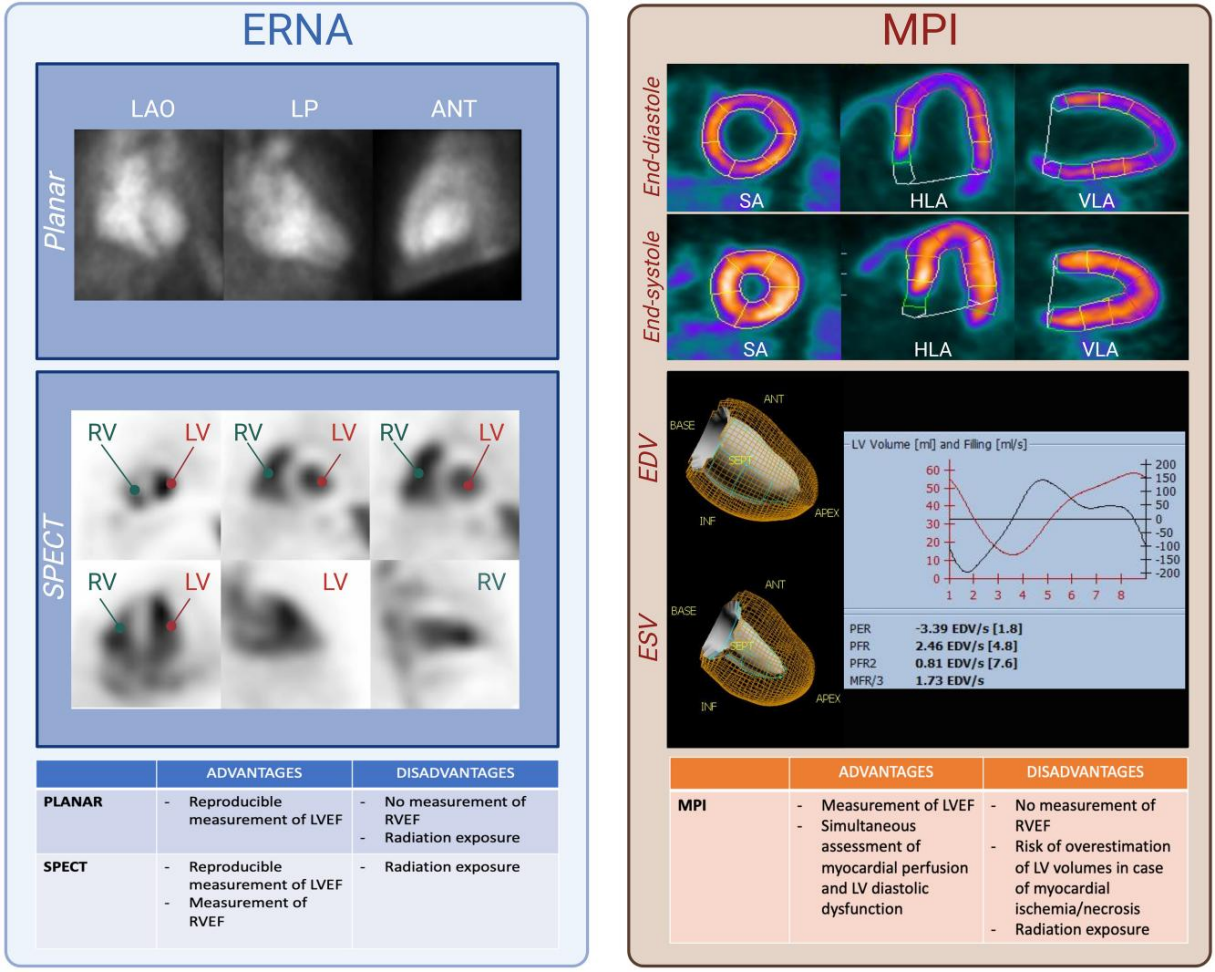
LV function assessment with nuclear cardiology. *Left panel*: ERNA techniques for LVEF assessment based on radiolabelled erythrocytes’ activity. Planar ERNA: end-diastolic and end-systolic LV volumes derived from LAO projections. Additional incidences include LP and anterior projections. SPECT ERNA: 3D reconstructions allowing LVEF/RVEF measurement. *Right panel*: NH_3_ PET MPI during end-diastole and end-systole enabling EDV/ESV estimation. Accurate volume measurement with MPI necessitates preserved myocardial perfusion. Diastolic (D) function can also be studied. Abbreviations: ANT, anterior; CHIP, clonal haematopoiesis of indeterminate potential; EDV, end-diastolic volume; ERNA, equilibrium radionuclide angiography; ESV, end-systolic volume; HLA, horizontal long axis; LAO, left anterior oblique; LP, left profile; LV, left ventricle; LVEF, left ventricular ejection fraction; MFR, mean filling rate during the first third of diastole; mL, millilitres; mL/s, millilitres per second; MPI, myocardial perfusion imaging; PER, peak ejection rate; NH_3_, ammonium; PET, positron emission tomography; PFR, peak filling rate; PFR/2, peak filling rate during the first half of diastole; RV, right ventricle; RVEF, right ventricular ejection fraction; SA, short axis; SPECT, single-photon emission computed tomography; VLA, vertical long axis.
